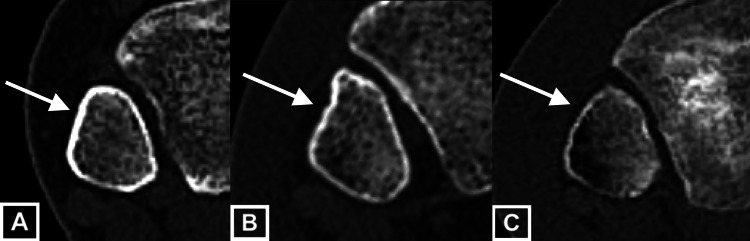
Fibular morphologies CT scan slices of different fibulas demonstrating (a) linear fibula morphology, (b) concave fibula morphology, and (c) convex fibula morphology
